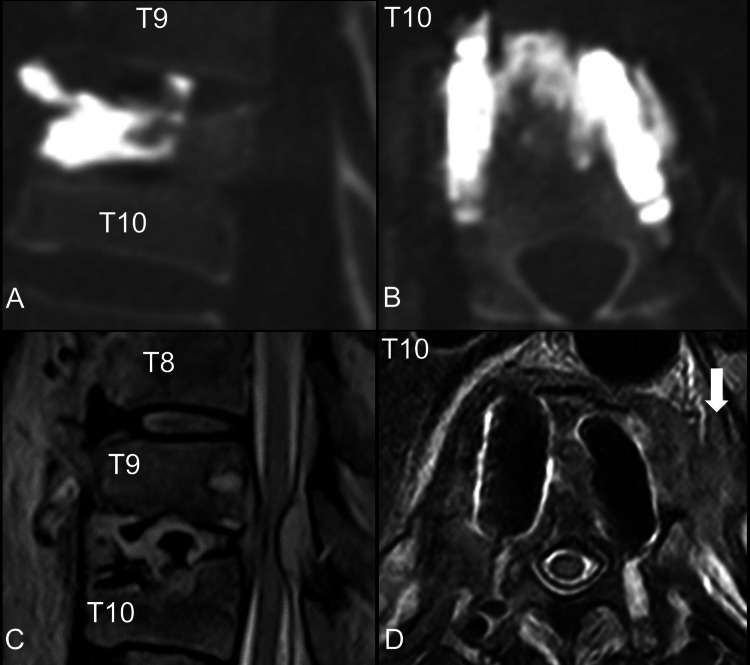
Post-vertebral augmentation images of the thoracic spine. (A) Computed tomography, bone window, sagittal view. (B) Computed tomography, bone window, axial view. (C) Magnetic resonance image, T2 without contrast, sagittal view, T10 level. (D) Magnetic resonance image, T2 without contrast, axial view, T10 level. Abnormal soft tissue was found in the paravertebral space (arrow).
